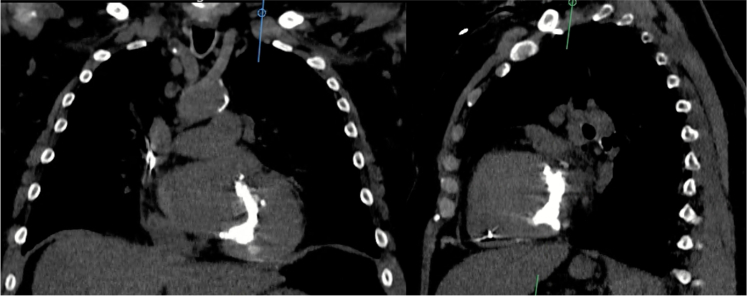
Chest computed tomography scan, coronal and sagittal views, demonstrating severe MAC.
